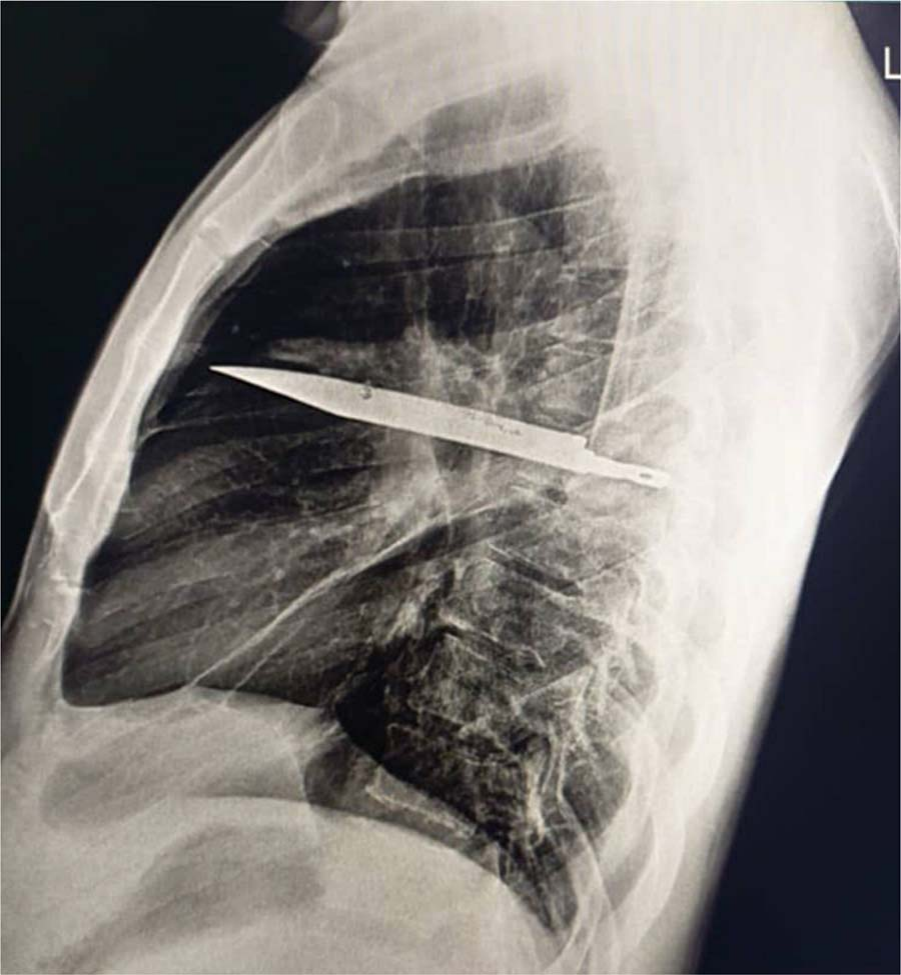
Lateral chest radiograph demonstrating a retained metallic object (knife) in the mid-thorax with surrounding opacification consistent with post-traumatic fibrosis following a stab wound.
